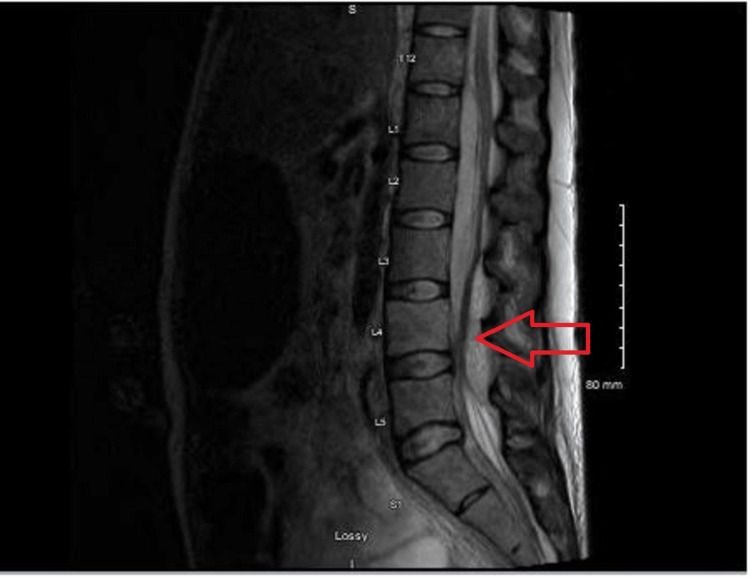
T2 sagittal view MRI of the lumbar spine. A large epidural abscess extended from T12 to L4, with the largest measurement of 11 × 22 mm at L1-2, causing severe central canal stenosis and posterior displacement of the thecal sac. Another large abscess was present from L4 to L5, measuring 11 × 10 mm, resulting in severe central canal stenosis and anterior displacement of the thecal sac.
